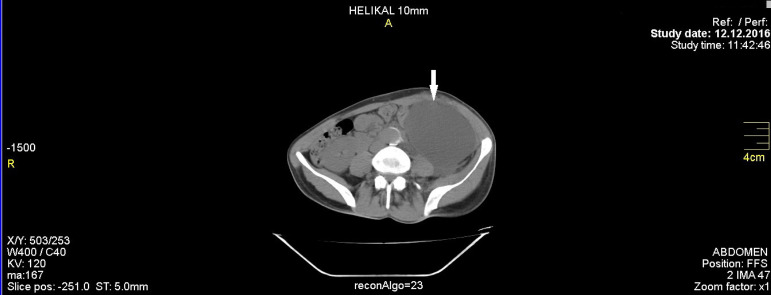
Postoperative thoracoabdominal computed tomography. Massive chylous fluid collection in the abdominal cavity.
